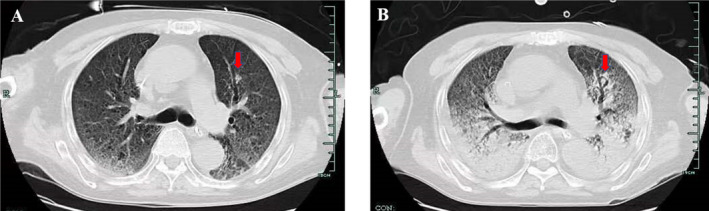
Lung CT findings of case 1. (A) The CT image was taken one day after the diagnosis of CAPA. It is showing diffuse ground glass shadow, interstitial lesions in both lungs and the small nodule in the left upper lung. (B) A follow‐up of CT after 1‐week showed that consolidation was aggravated; there was a cavity and crescent sign in the upper left.
